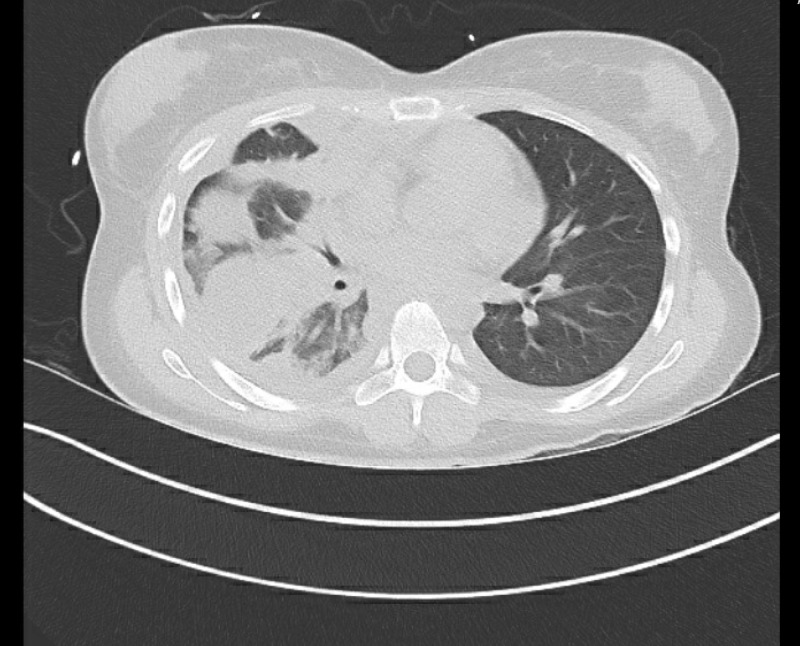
Computed tomographic scan done prior to video-assisted thoracoscopic surgery (VATS)
